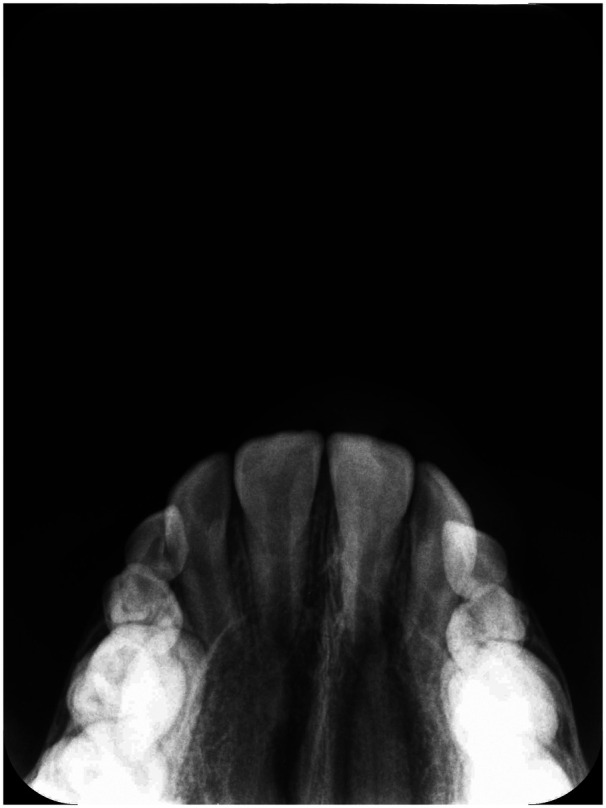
Occlusal radiograph at T0 in a patient treated with IPE.
